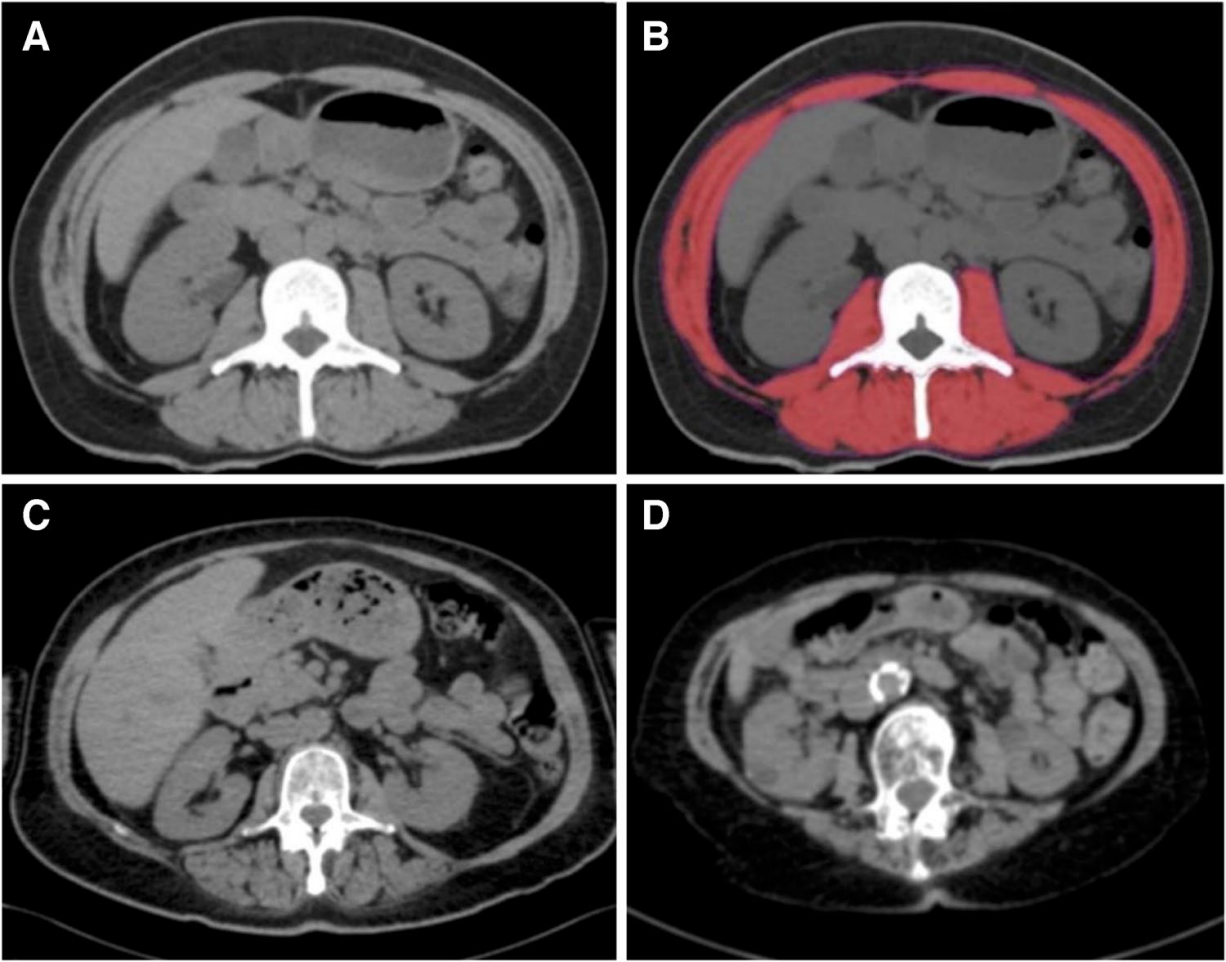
Cross-sectional CT images at the level of the third lumbar vertebra in patients with myosteatosis and sarcopenia. A Axial CT image at the level of the third lumbar vertebra identified for analysis. B Red area indicating segmentation of skeletal muscle. C Myosteatosis in a 64-year-old woman (MA: 19 HU). D Sarcopenia in a 72-year-old woman (SMI: 23 cm^2^/m^2^). Abbreviations: CT: Computed Tomography; HU: Hounsfield Units; MA: muscle attenuation; SMI: skeletal muscle index
